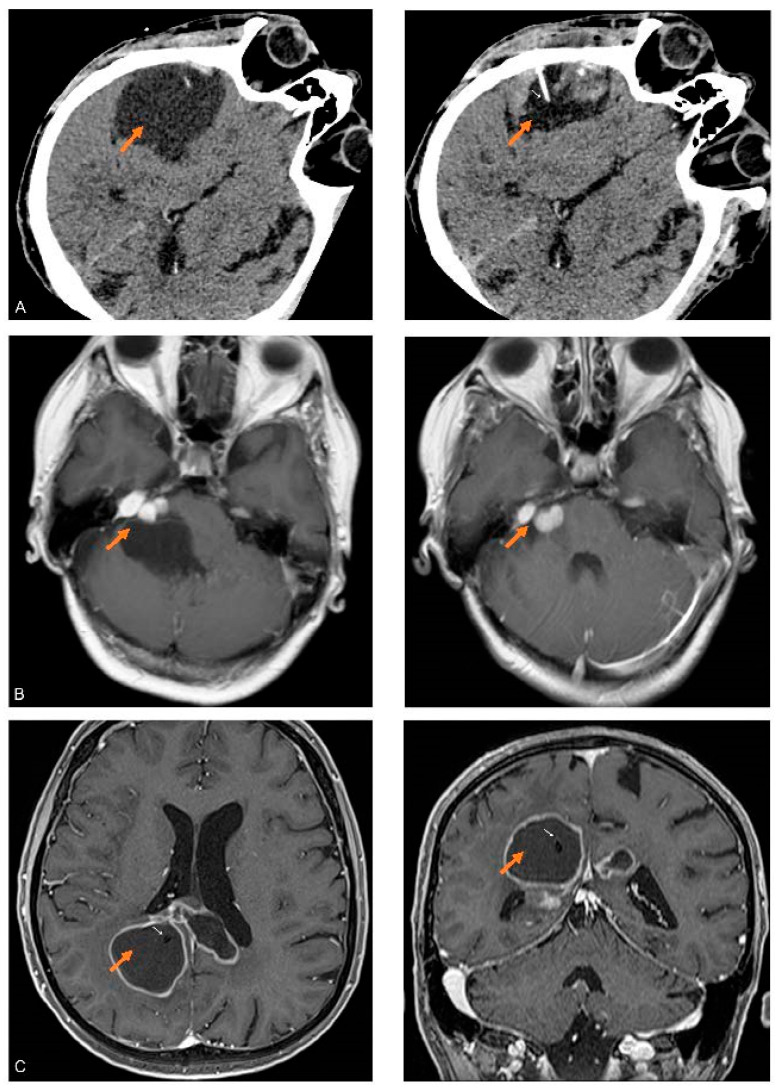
CT and MRI images of the cystic brain tumors studied: (A) Axial CT image of the SM obtained before (left) and after partial aspiration (right) of fluid in the cyst (orange arrows). In the right image, the needle (white arrow) used for the aspiration of the fluid is visible in the shrunken cystic cavity. (B) Axial MRI T1 weighed images with contrast, obtained before (left) and after (right) partial aspiration of fluid in the cyst (orange arrows) associated with the right-sided CS. (C) Axial and coronal MRI T1 weighed images with contrast of CG after the implantation of a catheter (white arrows) inside the largest cyst (orange arrows).
